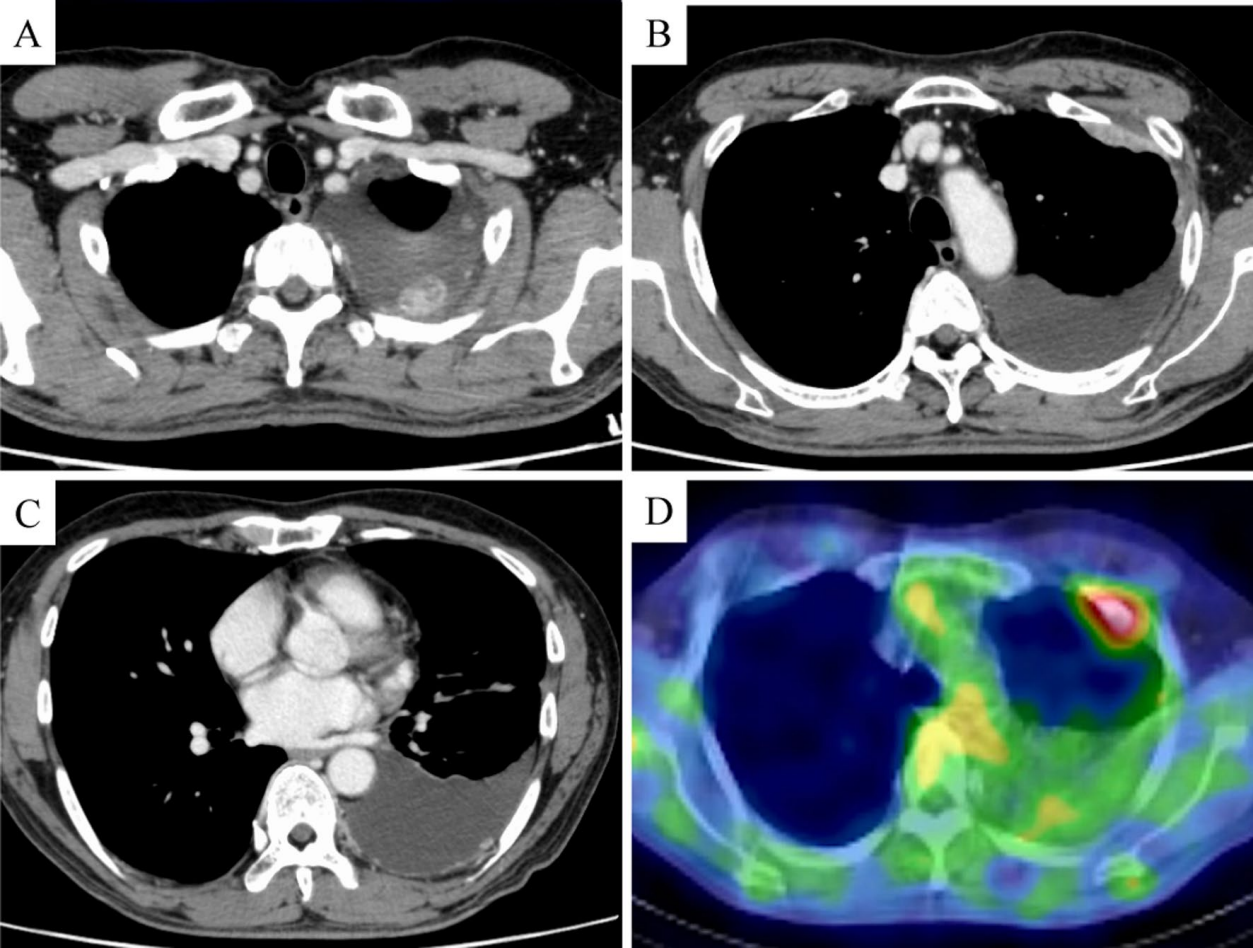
A–C Contrast-enhanced CT of the chest reveals pleural thickening and pleural nodular lesions with left pleural effusion. D PET–CT demonstrates markedly increased FDG uptake within the pleural nodular lesion. *CT* computed tomography, *PET–CT* positron emission tomography–computed tomography, *FDG* fluorodeoxyglucose
